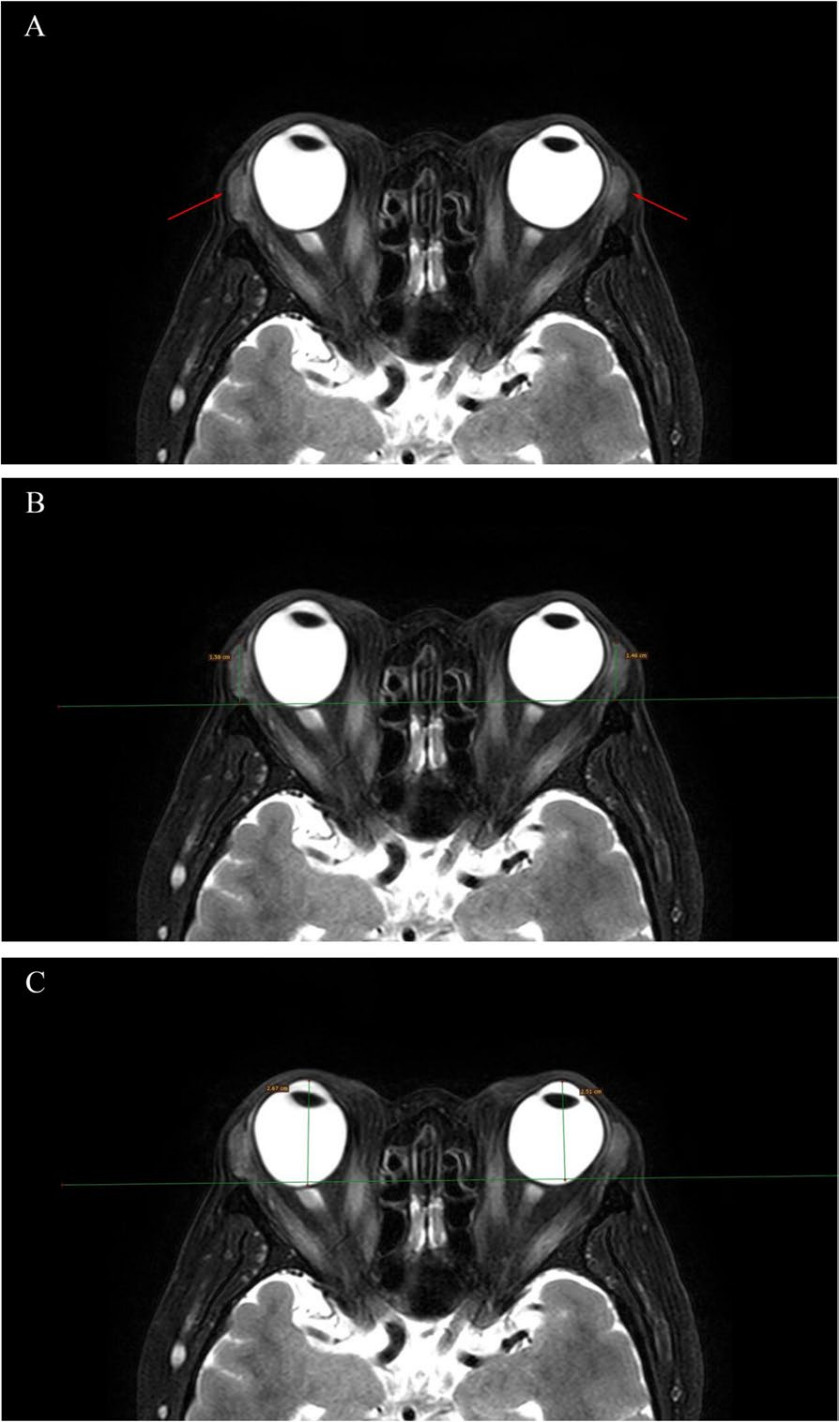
An axial 3-mm-thick T2-weighted fat suppressed axial image of a patient with thyroid-associated ophthalmopathy. The arrows in (A) show bilateral prolapse of the lacrimal glands. (B) Lacrimal gland prolapse, measured as the vertical distance from the top of the lacrimal gland to the interzygomatic line, is 15.1 mm on the right and 14.6 mm on the left. (C) Exophthalmos is measured as the vertical distance from the top of the cornea to the interzygomatic line
